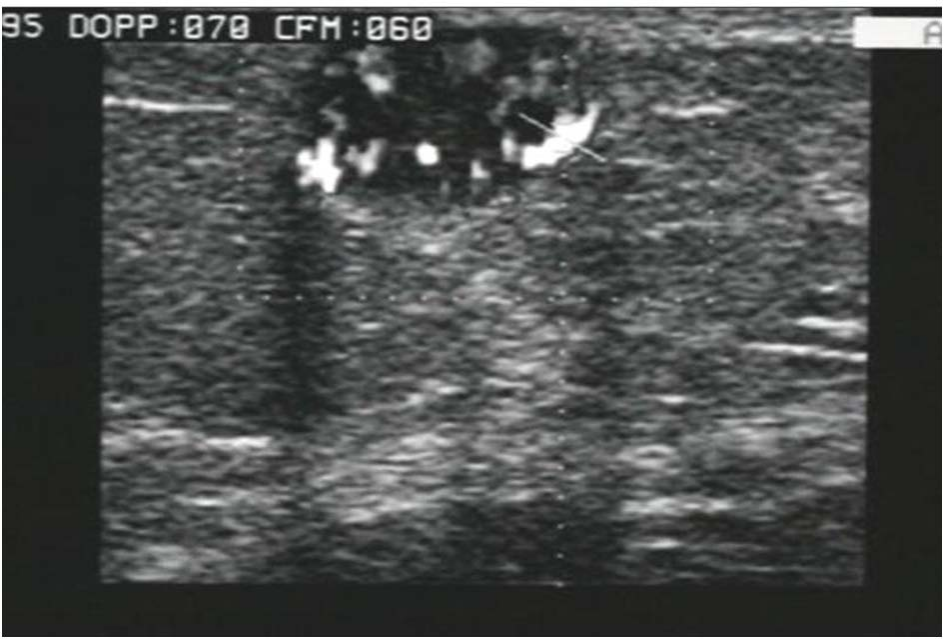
Pattern type 5: Pseudo-neoplastic, solid nodulation, hypoechogenic, not homogeneous, with irregular anterior contours, with signal with Colour and Power-Doppler.
